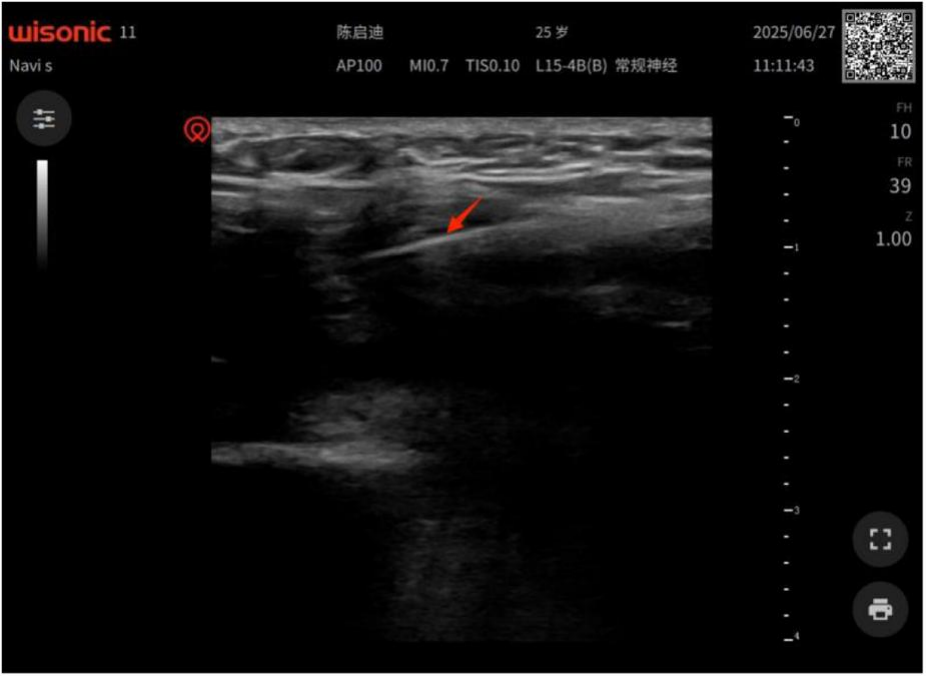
Under ultrasound: a strong echo of the needle tip is visible when the needle tip reaches the surface of the adhesive tissue.
